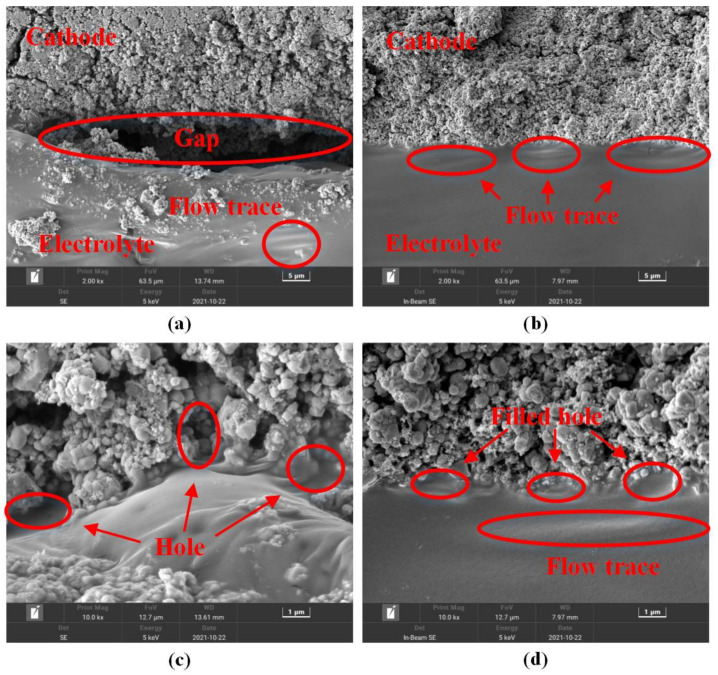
Electrolyte/cathode interface morphologies from: (a) Hot-pressed Group, 2000×; (b) Ultrasonic Group, 2000×; (c) Hot-pressed Group, 10,000×; (d) Ultrasonic Group, 10,000×.
